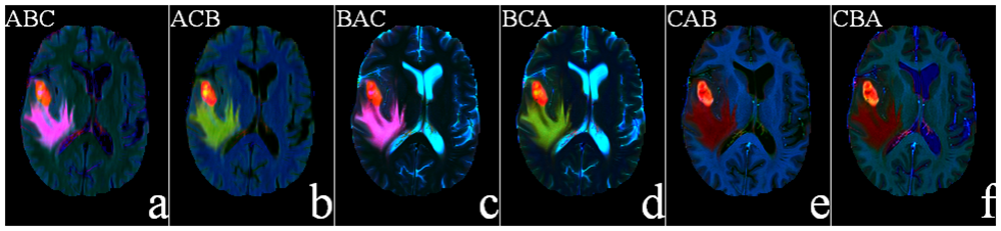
Six pseudo-colored (*L *a *b) converted brain MR image sequences, corresponding to the six pseudo-colored (*RGB*) MR sequences of Fig 3.
